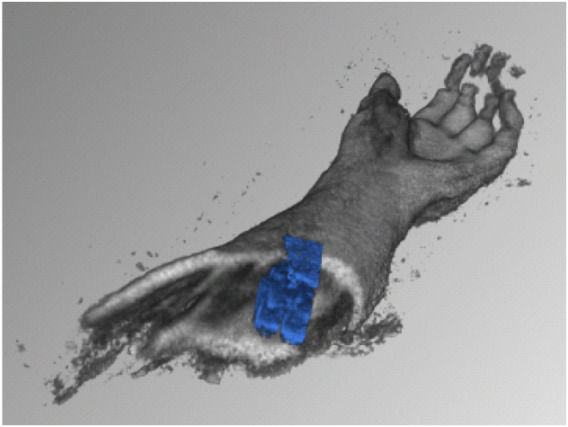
Early results of scanning a human forearm in a water tank, with fast automatic compounding of 3D ultrasound volumes [taken from Jauer et al. (2015)].
